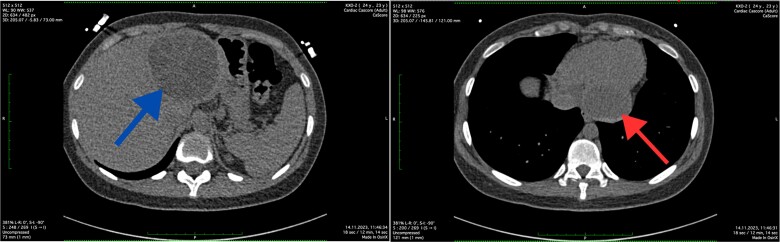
Axial CT scan showing the cystic lesion in the left lobe of the liver (blue arrow) and axial CT scan showing the cystic lesion in the left ventricle (red arrow).
